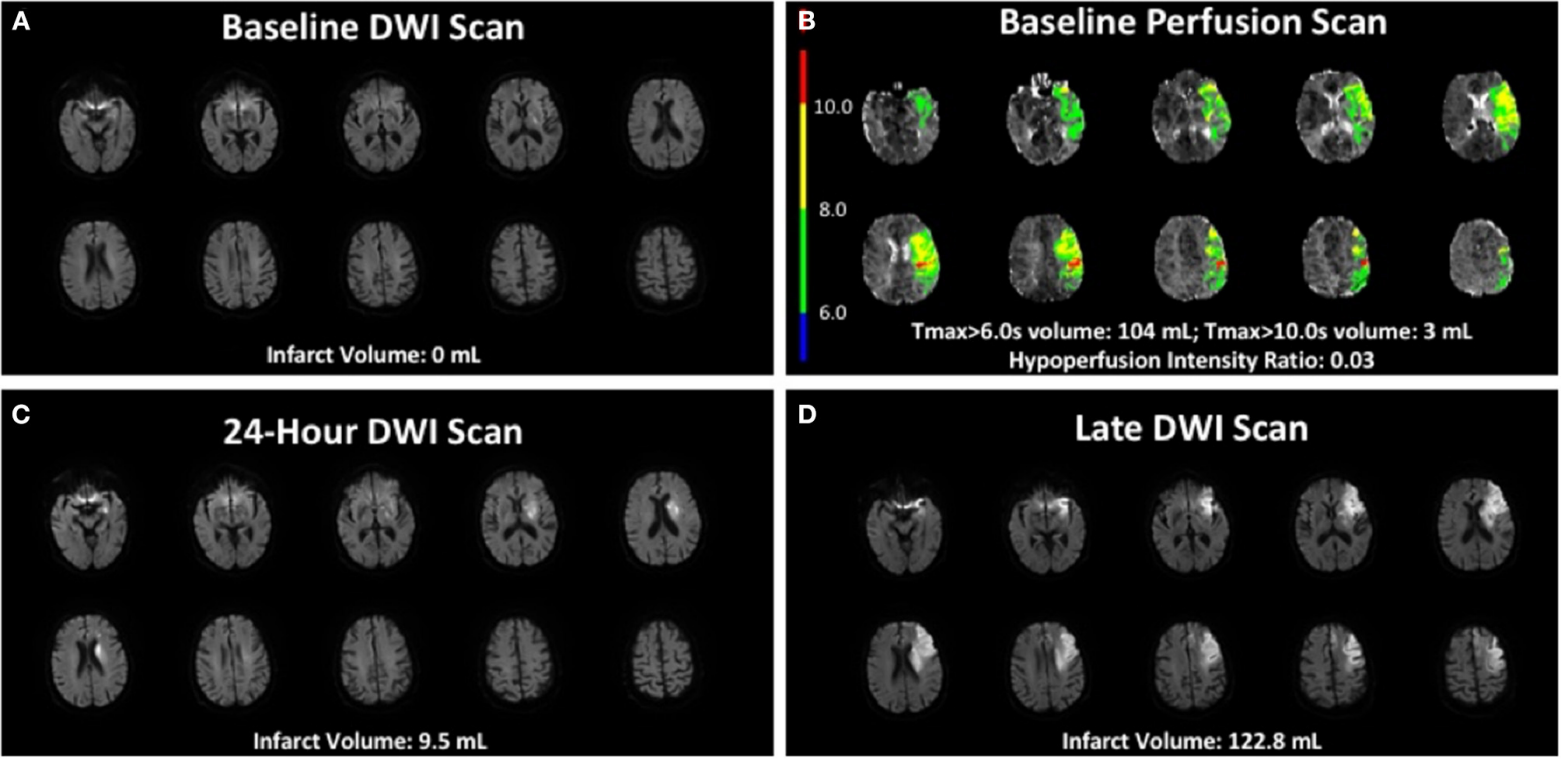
Case example of baseline DWI and perfusion scan, a 24-h DWI scan, and a late DWI scan. Seventy-eight-year-old patient with 12 h of symptoms prior to baseline MRI, with good baseline collaterals but who did not reperfuse with imaging demonstrating minimal early infarct growth and substantial late infarct growth. (A) Baseline MRI showed a 0 ml DWI infarct. (B) Baseline perfusion imaging showed good collaterals with a hypoperfusion intensity ratio of 0.03. (C) 24-h MRI showed a 9.5 ml DWI infarct. (D) Late MRI scan occurred 12 days after 24-h scan and showed a 122.8 ml DWI infarct volume.
